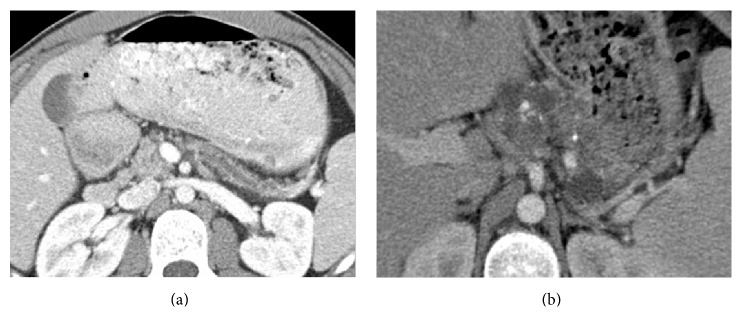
(a) Axial CT images of a 38-year-old male with cystic fibrosis. There is atrophy of the pancreas and duct irregularity. (b) Axial CT image of a 36-year-old female exhibiting pancreatic cysts, pancreatic calcification, and duct irregularity.
